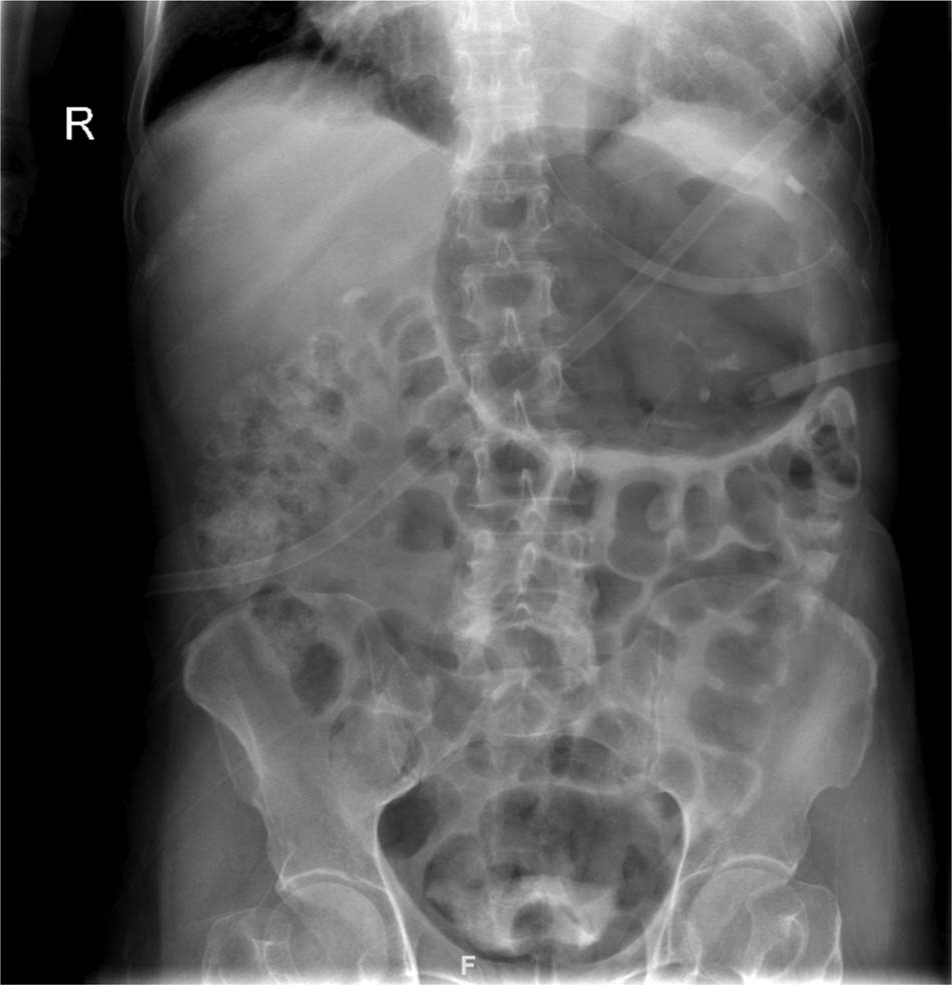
Abdominal radiograph in an anteroposterior (AP) projection demonstrates multiple dilated loops of small bowel with prominent air-fluid levels. The large bowel appears decompressed. A nasogastric tube is seen *in situ*, terminating in the stomach. No evidence of free intraperitoneal air is identified to suggest perforation. No radiopaque foreign bodies or mass lesions are visible.
